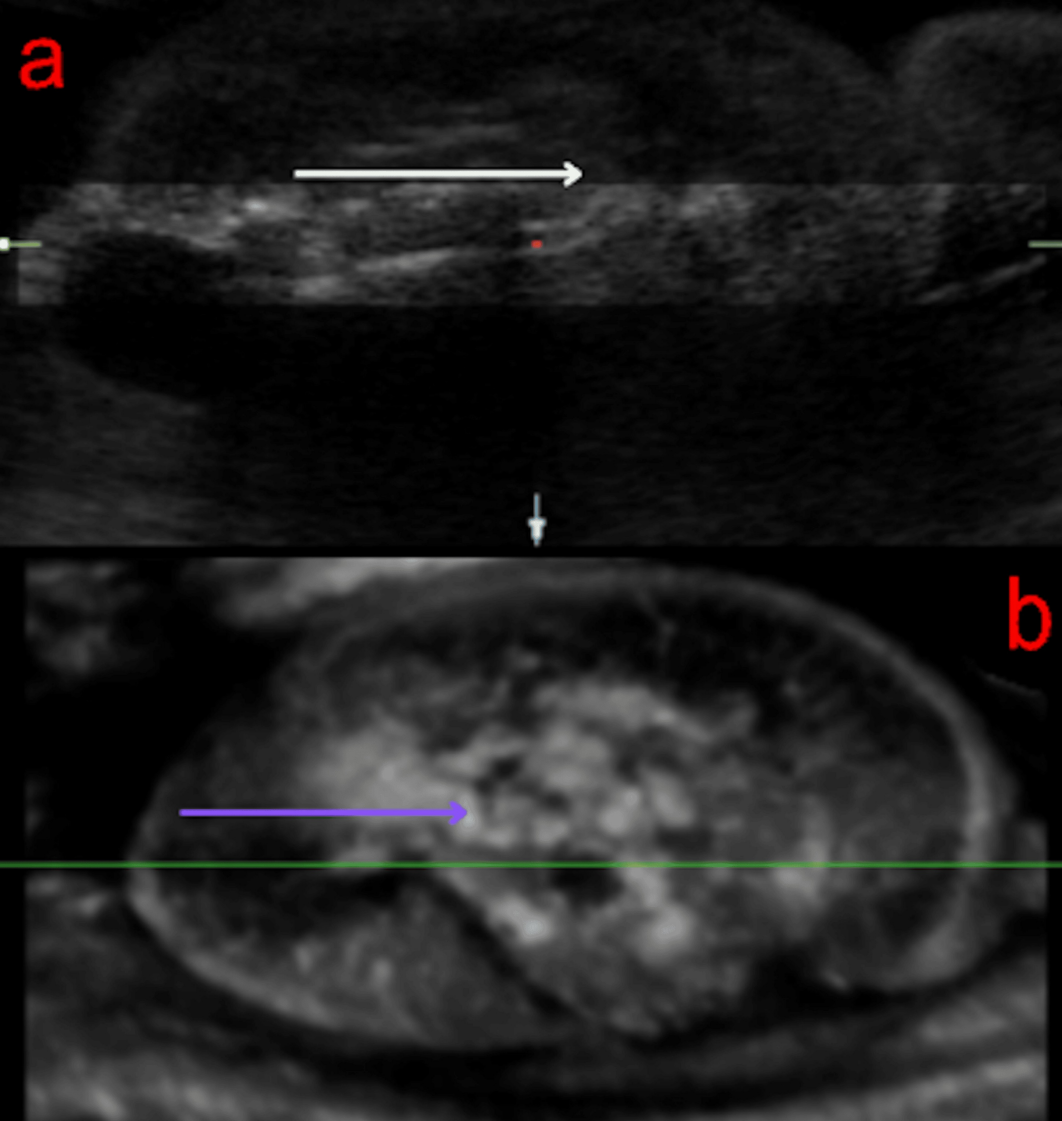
Three-dimensional multiplanar ultrasound rendering of acardius acormus. All body structures appear underdeveloped and disorganized, as indicated by the white arrow in panel (a) and the purple arrow in panel (b).
